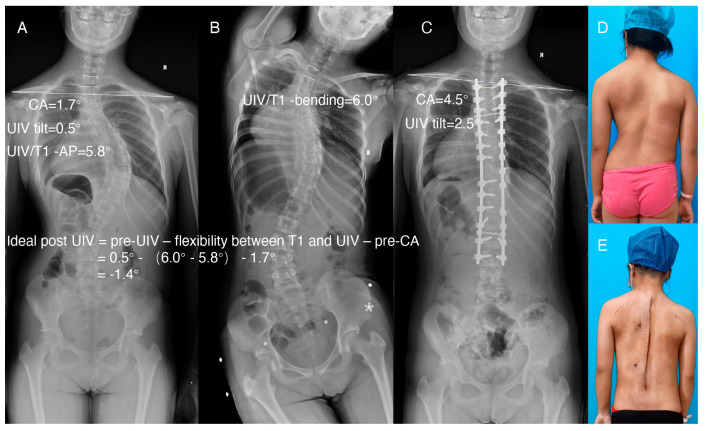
Representative LSI case in the retrospective study. (A) AP radiograph of the entire spine preoperatively. (B) Right bending. (C) AP radiograph of the entire spine postoperatively. (D,E) Clinical images of the same patient preoperatively and postoperatively. The ideal postoperative UIV tilt, calculated by the formula using the parameters measured in A and B, was −1.4 degrees. The actual postoperative UIV tilt was 2.5 degrees, and the postoperative CA tilt was 4.5 degrees.
